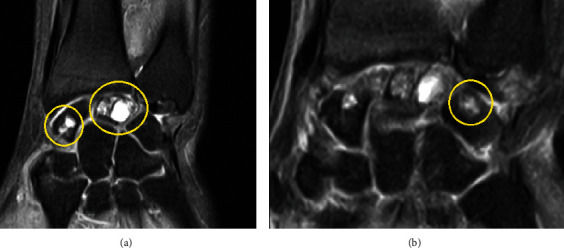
MRI of the left wrist showing IGC (from (a) to (b)) in the scaphoid, lunate (a), and triquetrum (b).
